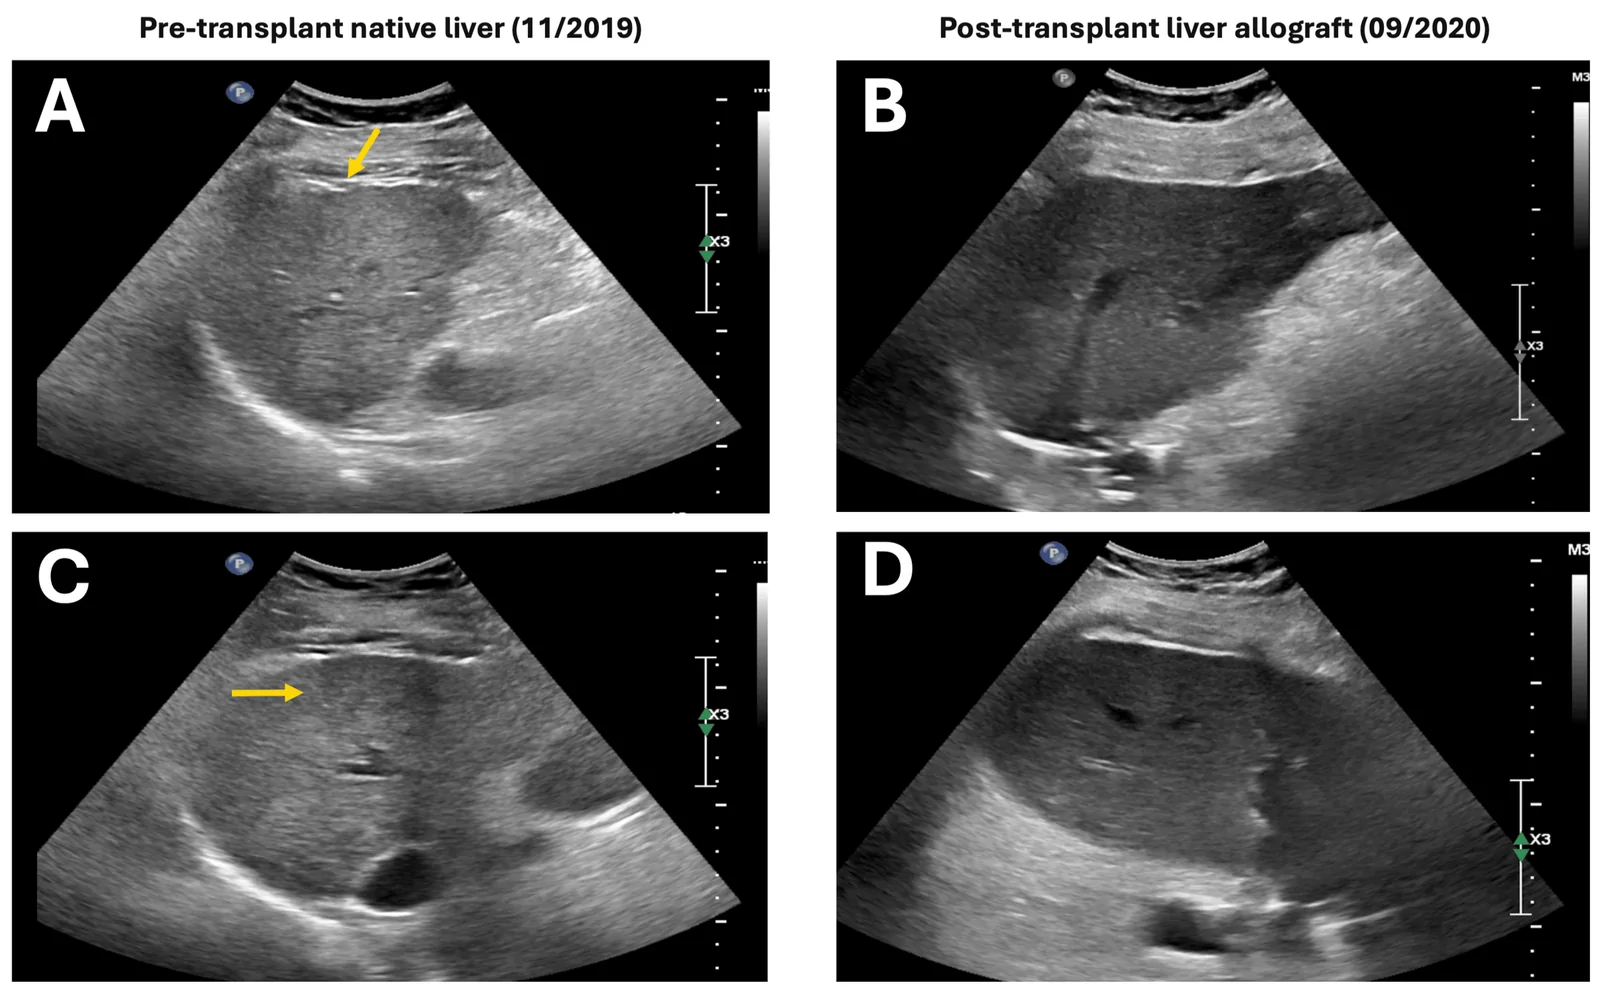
Right upper quadrant ultrasound of the pre-transplant cirrhotic native liver and post-transplant hepatic allograft (A) Pre-transplant longitudinal view of the native liver demonstrating nodular hepatic contour (arrow). (B) Post-transplant longitudinal view of the hepatic allograft demonstrating unremarkable morphology, parenchyma, and contour. (C) Pre-transplant transverse view demonstrating heterogeneous, coarsened hepatic parenchyma (arrow). (D) Post-transplant transverse view of the hepatic allograft demonstrating unremarkable morphology, parenchyma, and contour.
